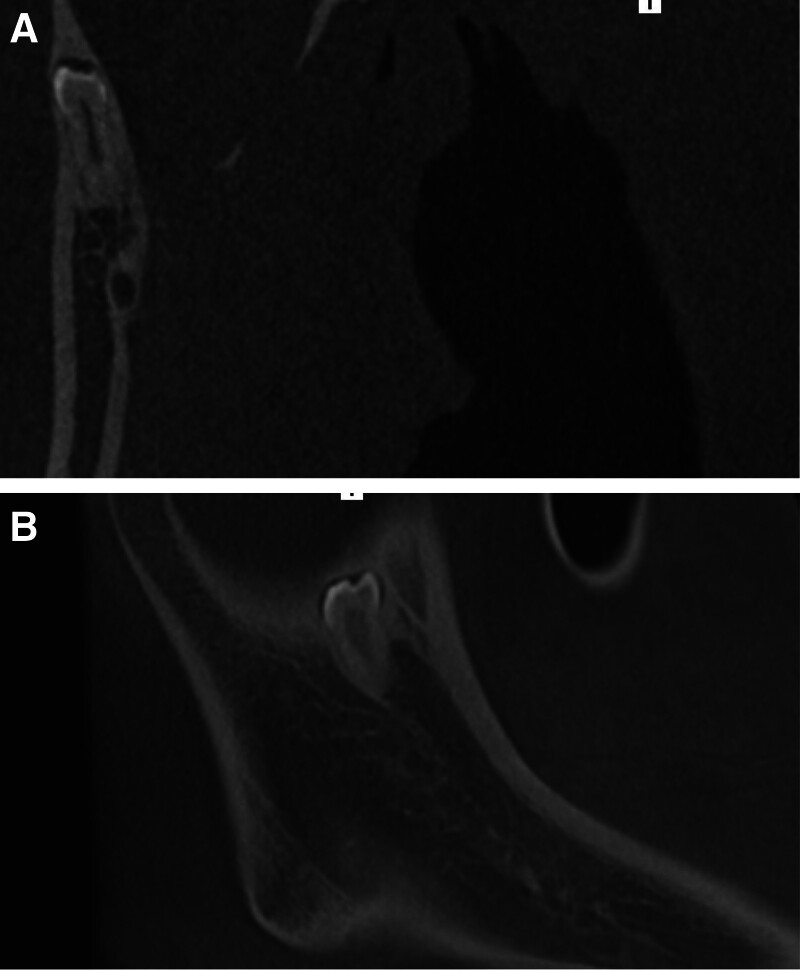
Imaging and dental clinical examination. (A and B) From the view of the coronal and sagittal position of CBCT. CBCT = cone beam computer tomography.
